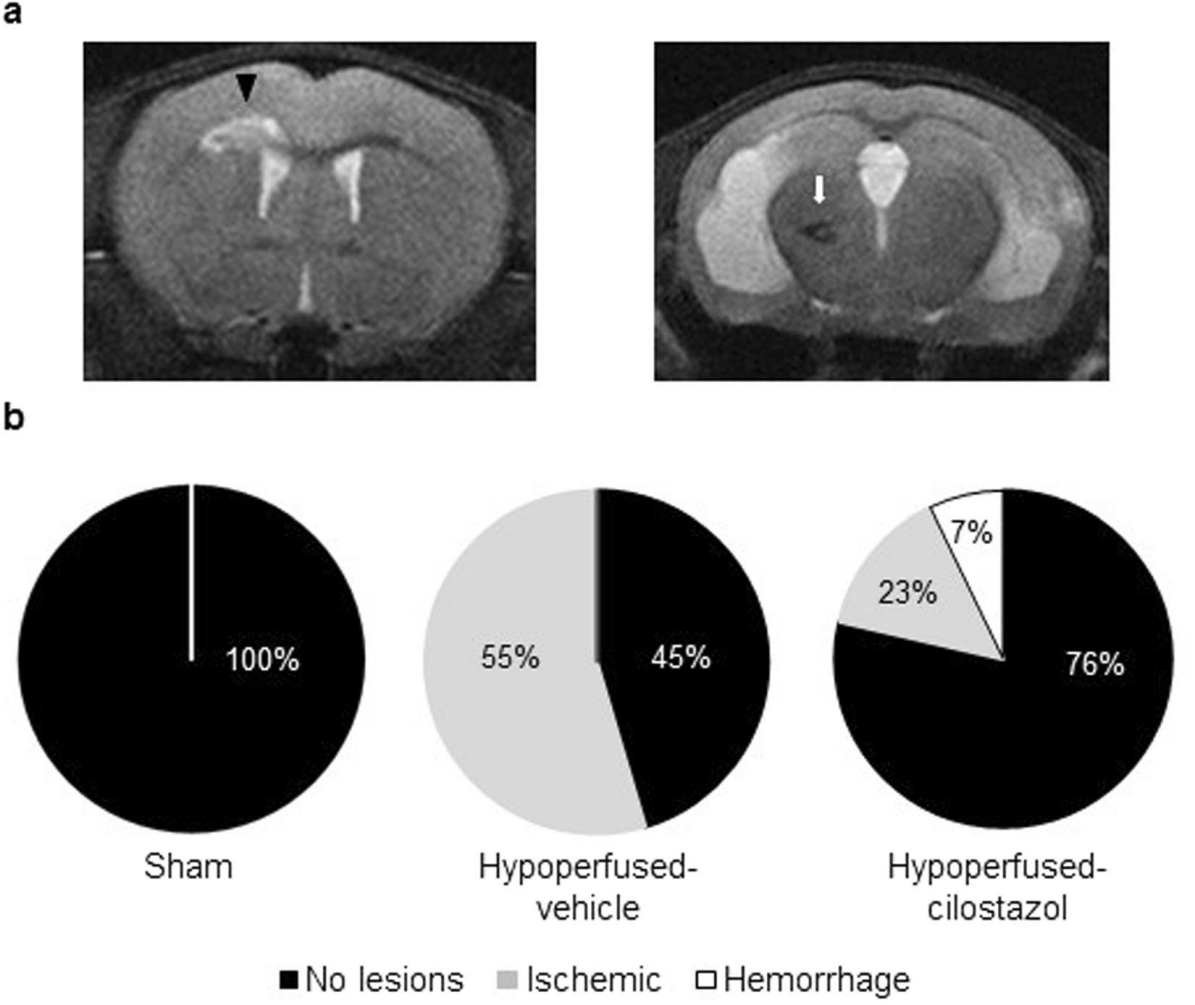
Cilostazol reduced stroke injury. (a) Representative images of T2-MRI detecting the ischemic (arrow head) and hemorrhagic (arrow) lesions. (b) MRI analysis showed that cilostazol remarkably decreased the number of the ischemic stroke lesions after chronic hypoperfuson.
